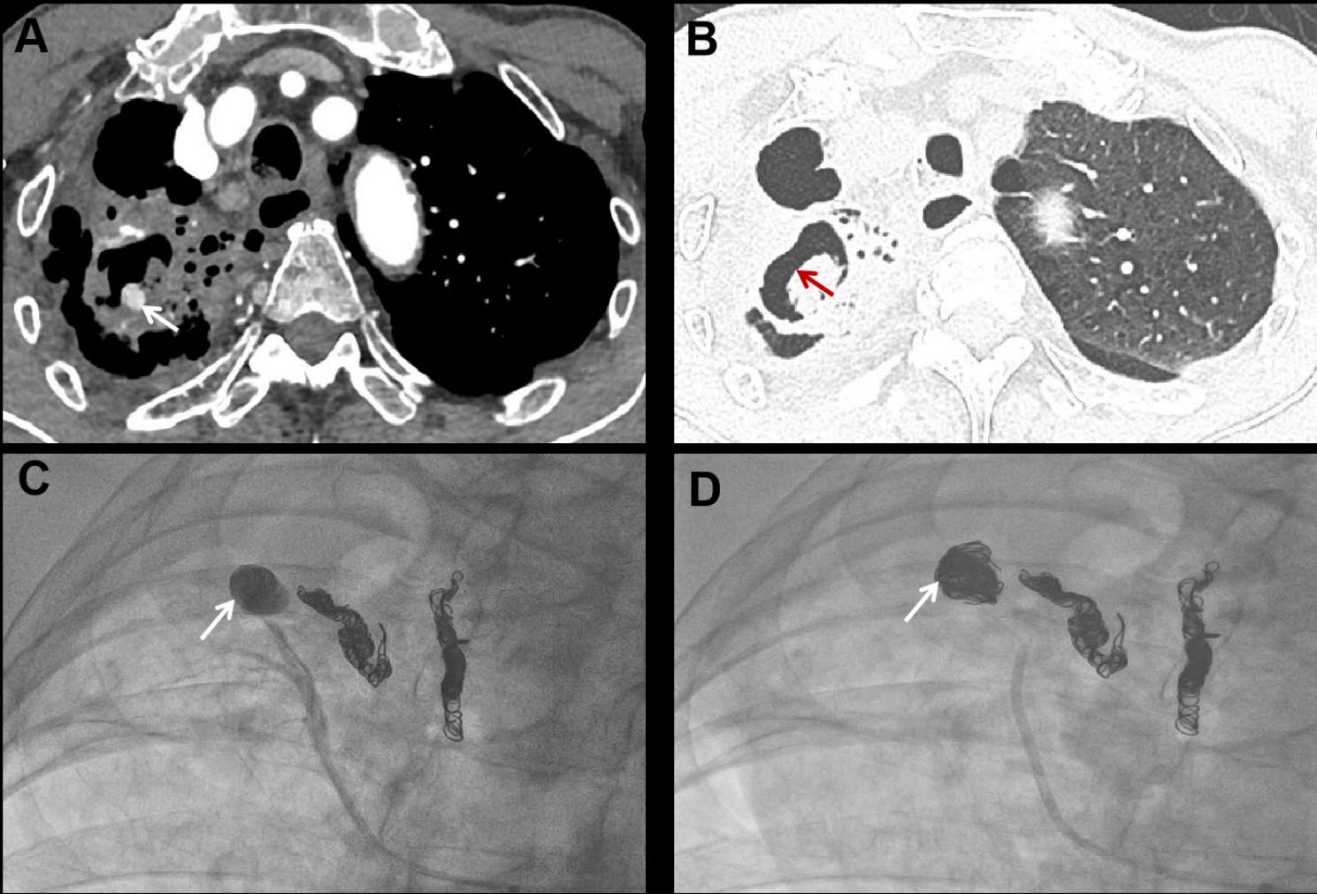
Representative images of Aspergillus infection combined with pulmonary artery pseudoaneurysm. A 48-year-old male was admitted with massive hemoptysis. Sputum culture confirmed aspergillus infection. Bronchial artery embolization was performed six months ago due to hemoptysis. (A) The axial enhanced CT scan reveals a PAP (arrow) located in the segmental pulmonary artery of the right upper lobe. The PAP is accompanied by cavitary lesions; (B) The axial enhanced CT scan displayed a crescent-shaped cavity (red arrow) in the right upper lobe, indicating a typical imaging manifestation of aspergillus infection; (C) Selective subsegmental pulmonary artery angiography confirmed the PAP (arrow); (D) The aneurysmal sac (arrow) was embolized with coils
